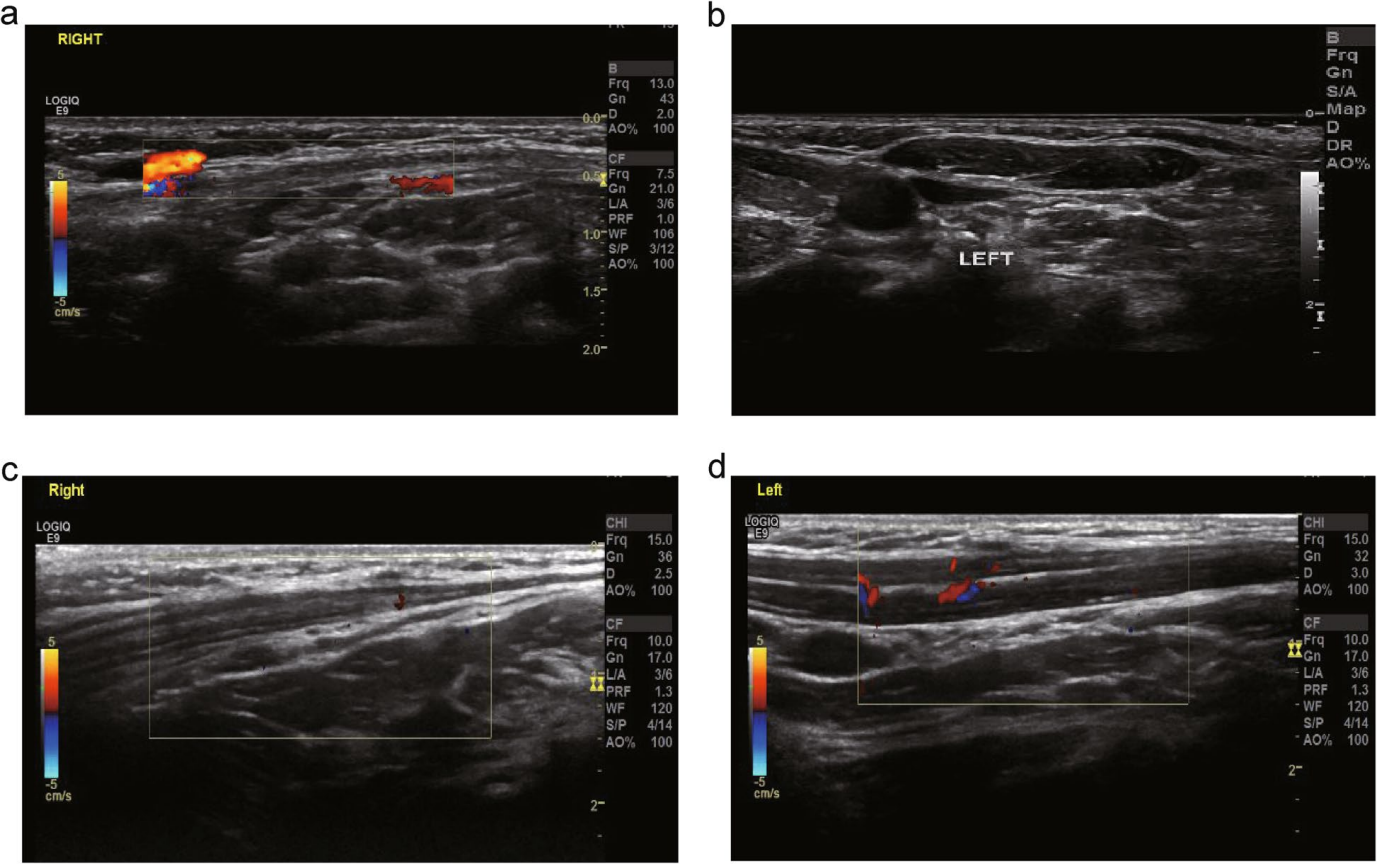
SCM ultrasound results of short-term observation (more than one year) in children with atrophy type. a At the first visit: diffuse thinning of the SCM on the affected side, mainly the sternal bundle, poor internal echo, clear muscle texture, and the thinnest part in the middle and the lower segment is about 1.5 mm. b At the first visit: the echo of the SCM on the healthy side is well-proportioned, and the thickness of the upper, middle and lower segments are 4.6 mm, 4.7 mm, and 4.7 mm, respectively. c One year later: the SCM of the affected side is diffusely thinned, mainly the sternal tract, the echo is uneven, and the muscle texture is still evident. The thickness of the upper, middle, and lower segments are 4.3 mm, 2.8 mm, and 1.7 mm, respectively. d Reexamination after one year: the SCM echo of the healthy side is uniform, and the thickness of the upper, middle, and lower segments are 4.5 mm, 4.6 mm, and 4.6 mm, respectively
